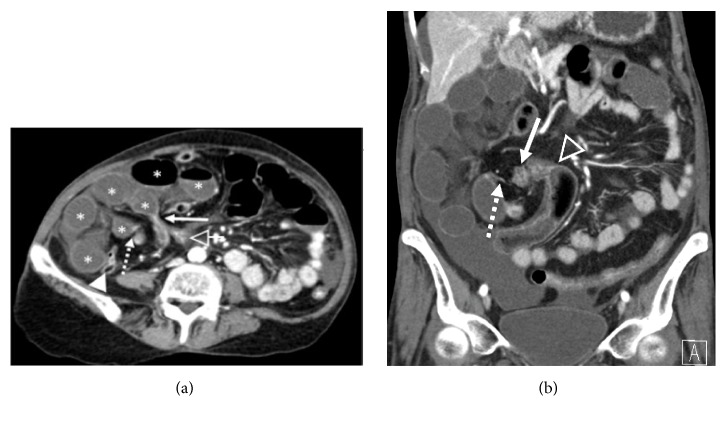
Contrast enhanced CT of the abdomen and pelvis demonstrating closed loop obstruction in the right lower quadrant. (a) Axial CECT demonstrating a proximal transition point within the proximal ileum (dashed arrow) and a nearby distal transition point within the distal ileum (solid arrow). The dilated bowel loops (*∗*) are anterolateral to the cecum (open arrowhead) and anterior to the ascending colon (closed arrowhead). (b) Coronal CECT image demonstrating multiple dilated small bowel loops confined to the right abdomen. Note the diminished enhancement of the dilated small bowel loops.
